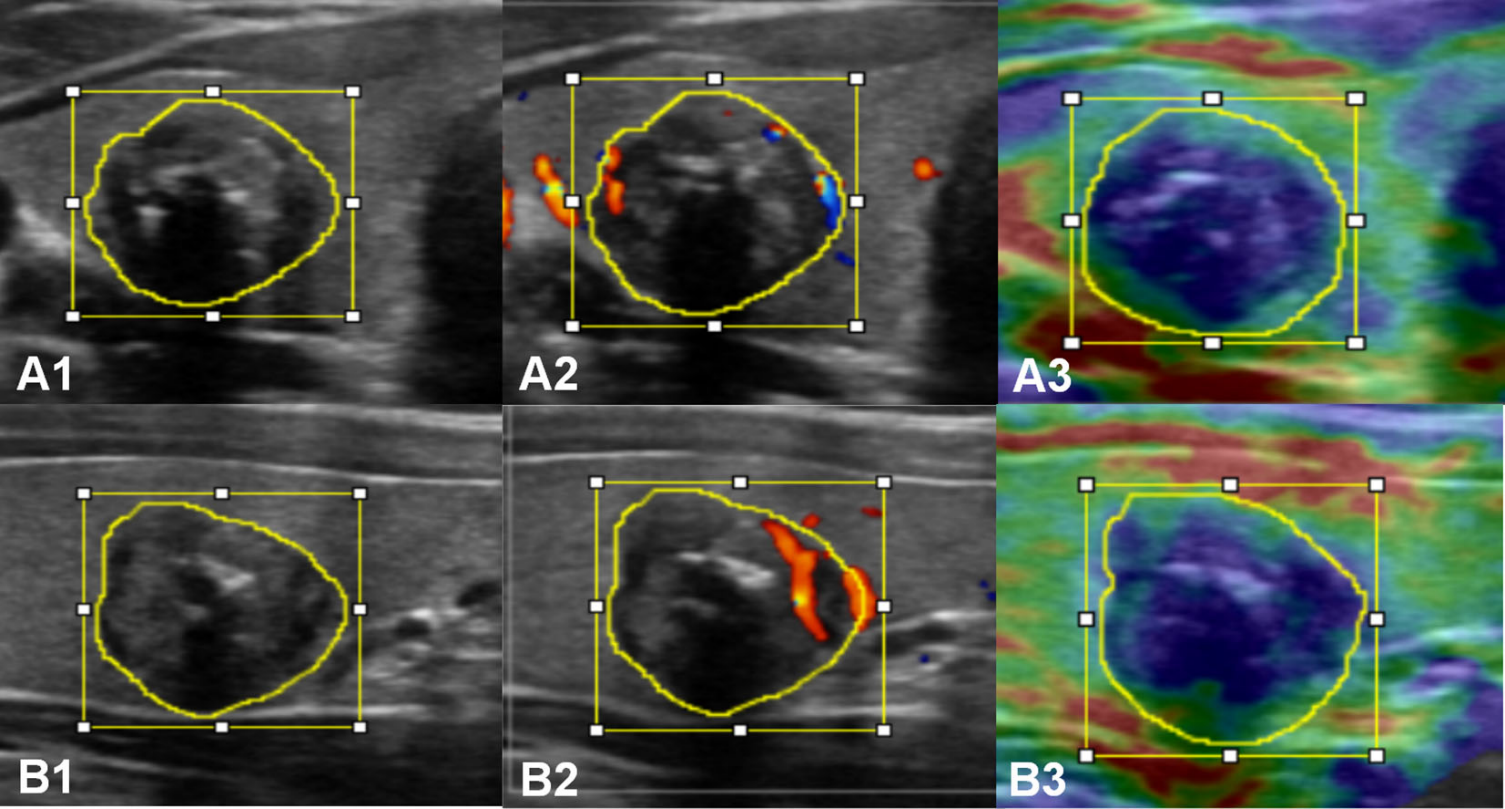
Multimodal ultrasound image ROI annotation of a primary PTC in the transverse (A) and longitudinal (B) sections. A1 and B1, grayscale; A2 and B2, CDFI; A3 and B3, strain elastography.
